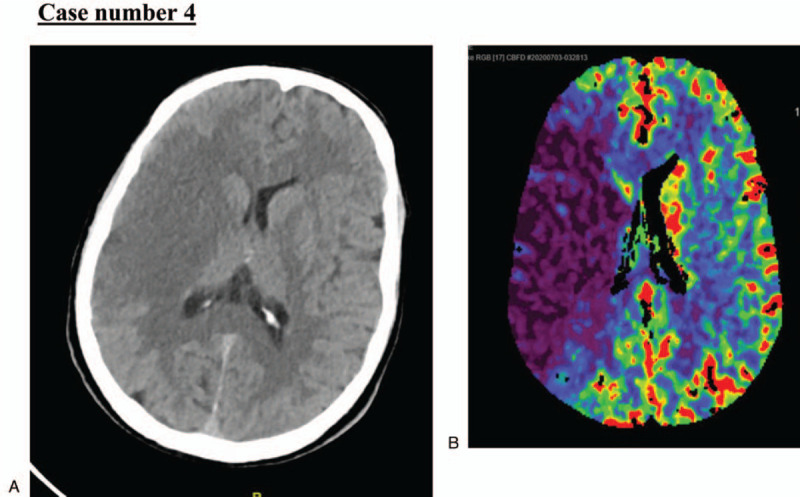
A. Plain computed tomography (CT) brain demonstrated loss of the right insular ribbon with hyperdense middle cerebral artery (MCA) sign. B. CT perfusion showed decreased cerebral flow and volume representing core infarcts with lack of areas of penumbra.
